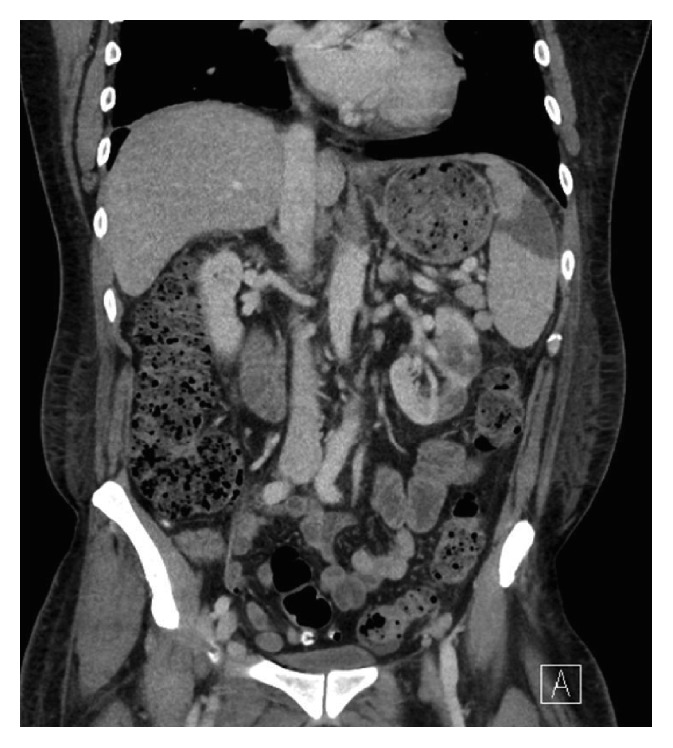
Computed tomography scan of chest/abdomen/pelvis demonstrating splenic and left renal infarction.
